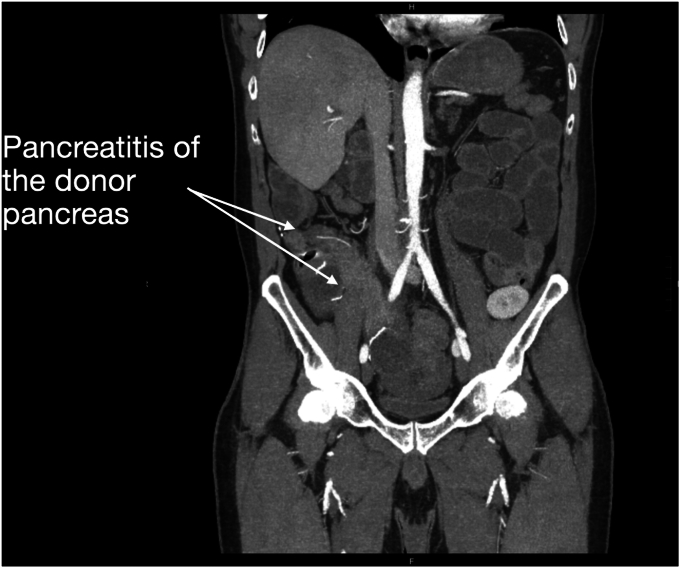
Intravenous contrast-enhanced computed tomography abdomen-pelvis acute interstitial pancreatitis of the donor pancreas with peripancreatic fat stranding. No pancreatic ductal dilation is seen.
